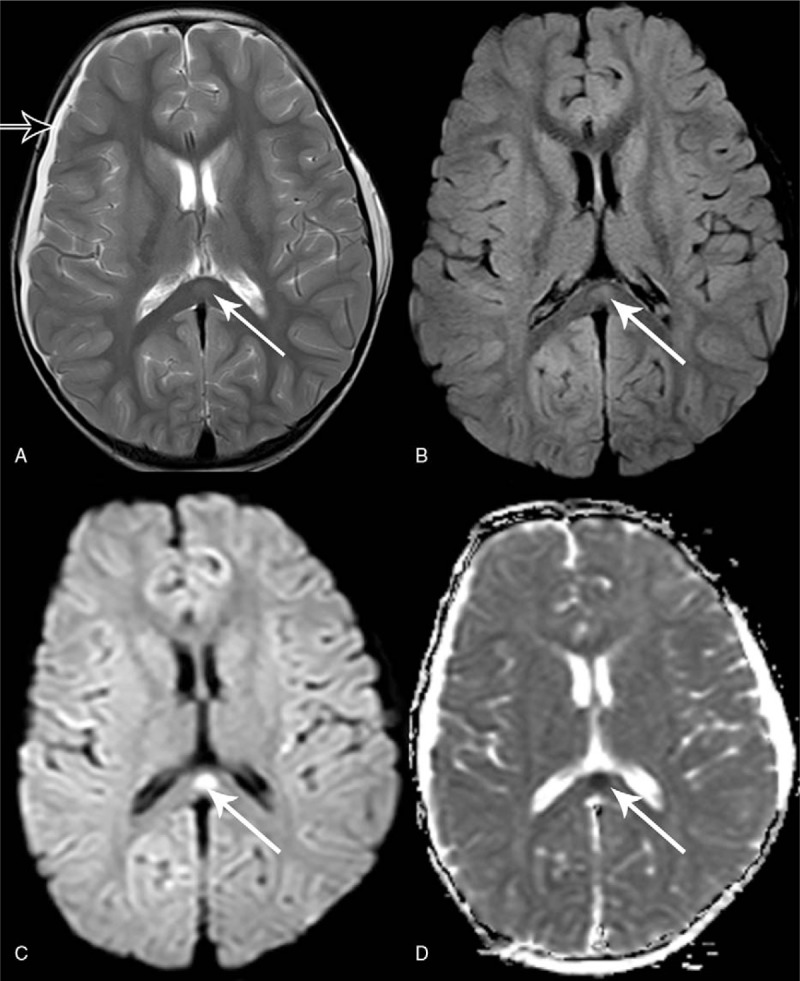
Cranial MRI showing right subdural effusion on T2 (A) and focal hyperintensity signal in the SCC on T2 (A), FLAIR (B), DWI (C), and diffusion restriction on ADC mapping (D). Hollow arrow showed right subdural effusion in (A). White arrows showed the lesion in the SCC on different images in (A, B, C, D).
